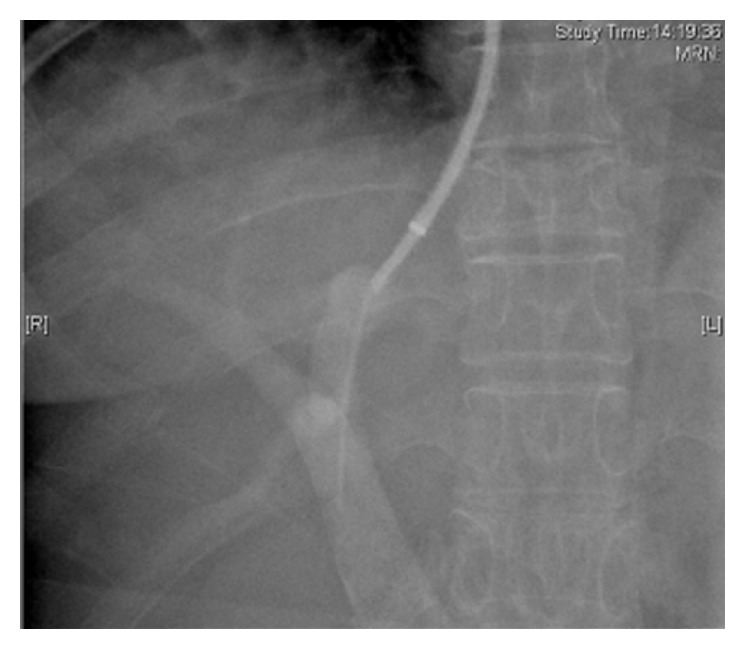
Portal venous blood samples were taken under guidance of X-rays.
